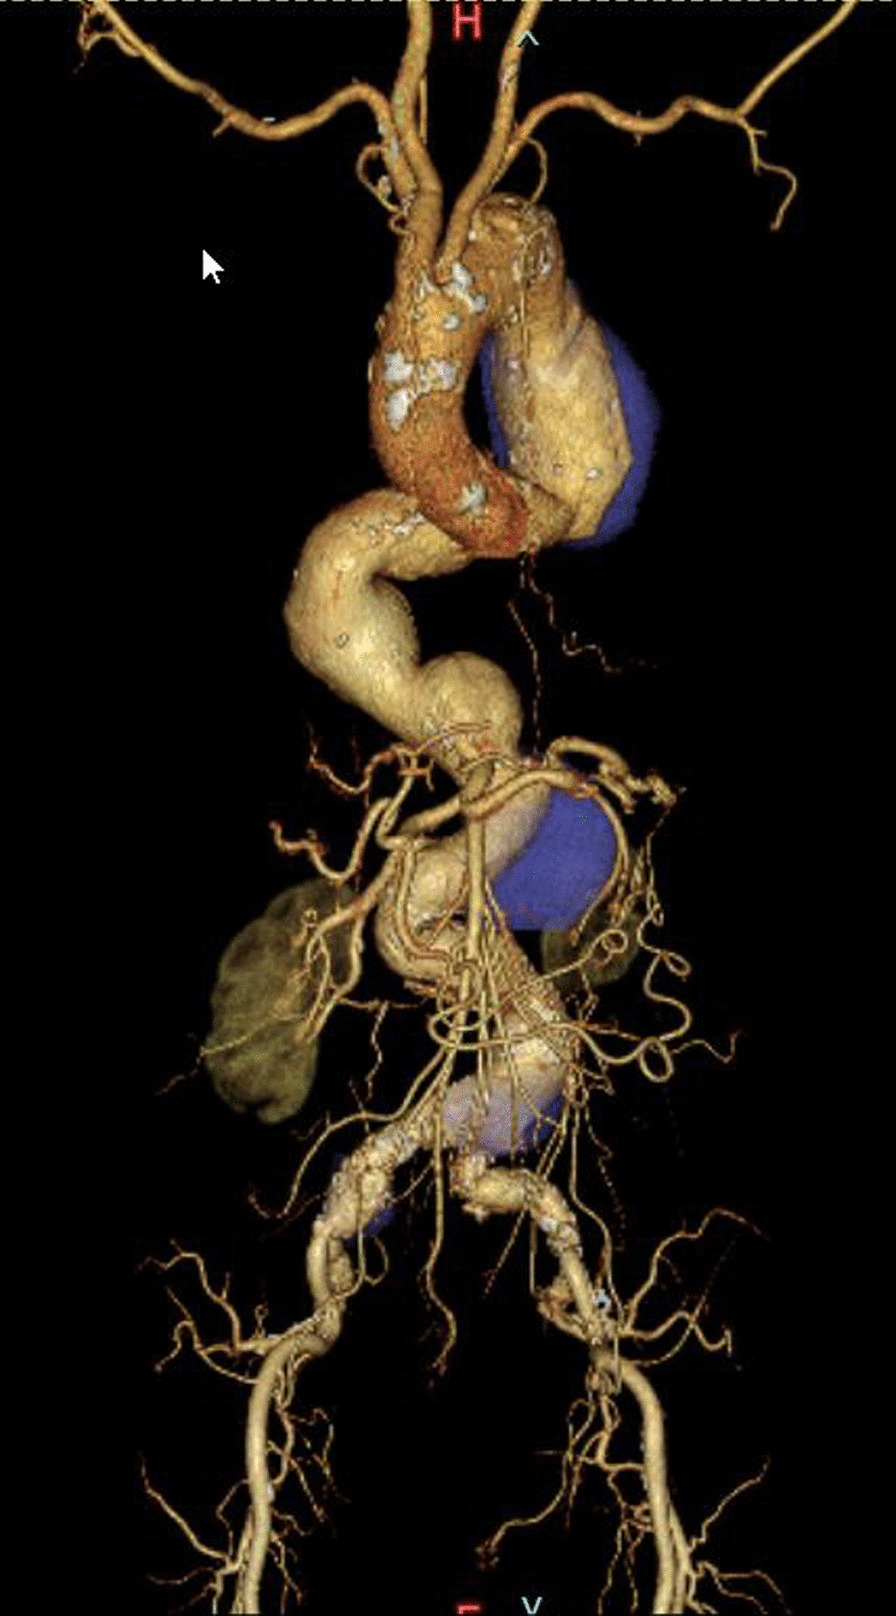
Preoperative computed tomography image. Computed tomography revealed a descending thoracic aortic aneurysm (65 mm in diameter), a thoracoabdominal aneurysm (54 mm in diameter), and an abdominal aortic aneurysm (49 mm in diameter)
